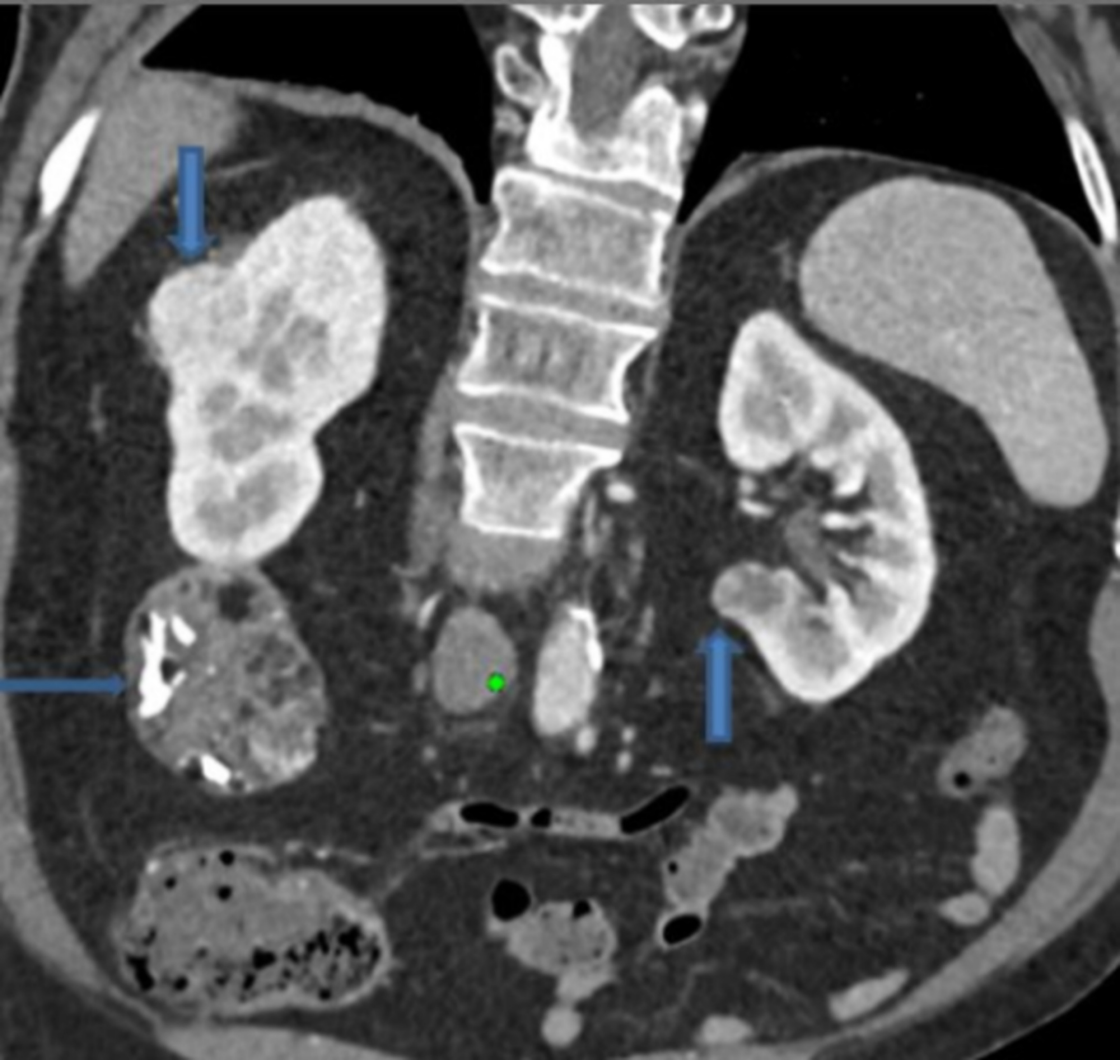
Contrast-enhanced CT scan of the abdomen and pelvis An oblique coronal view demonstrates all three lesions in the kidney. The one in the lower pole of the right kidney is the largest.
